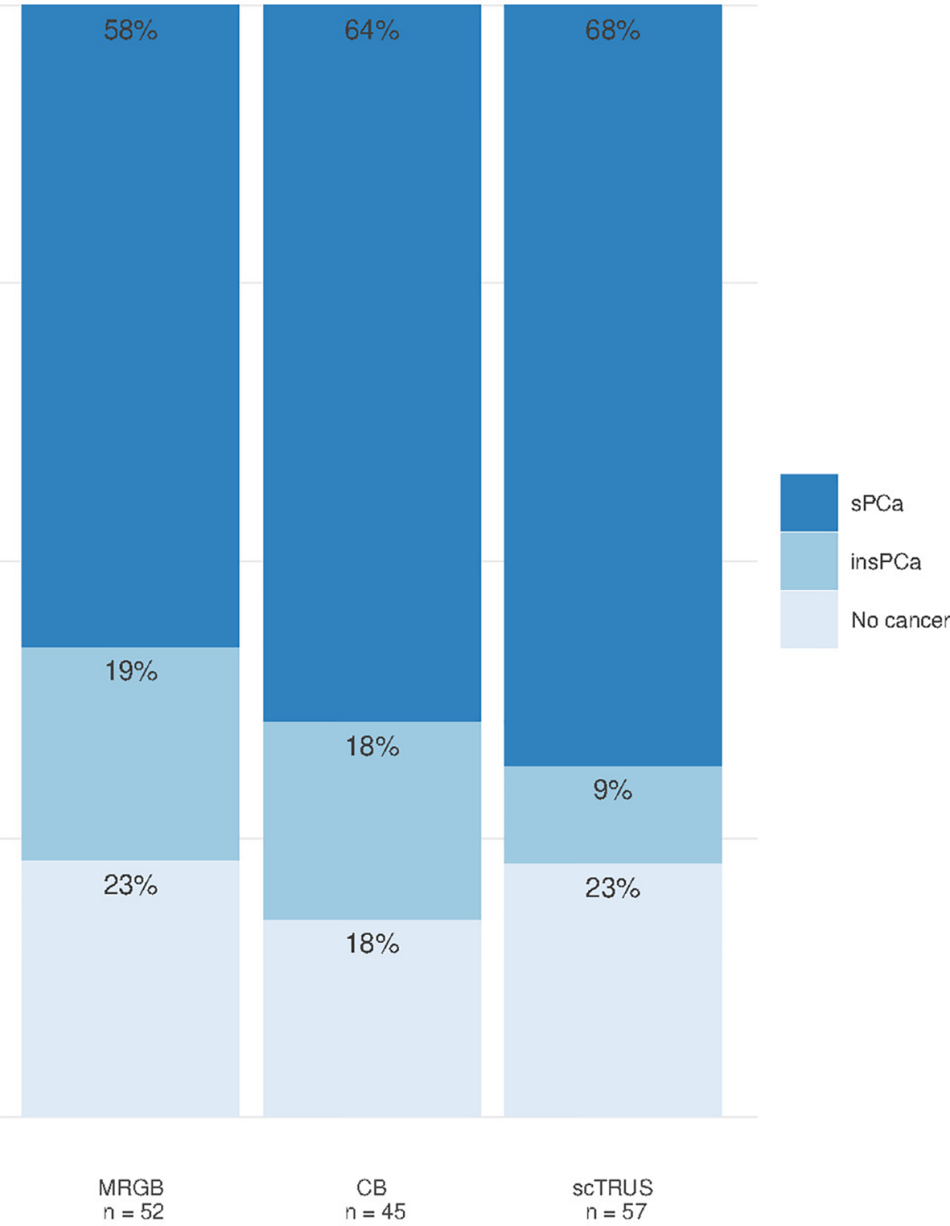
Detection rates of prostate cancer for MRGB, combined biopsy, and cognitive targeted TRUS. MRGB, MR-guided in-bore biopsy. CB, combined biopsy (MRGB with subsequent cognitive targeted TRUS with additional systematic biopsy cores); scTRUS, cognitive targeted transrectal ultrasound-guided biopsy with additional systematic biopsy cores; sPCa, significant prostate cancer; insPCa, insignificant prostate cancer.
